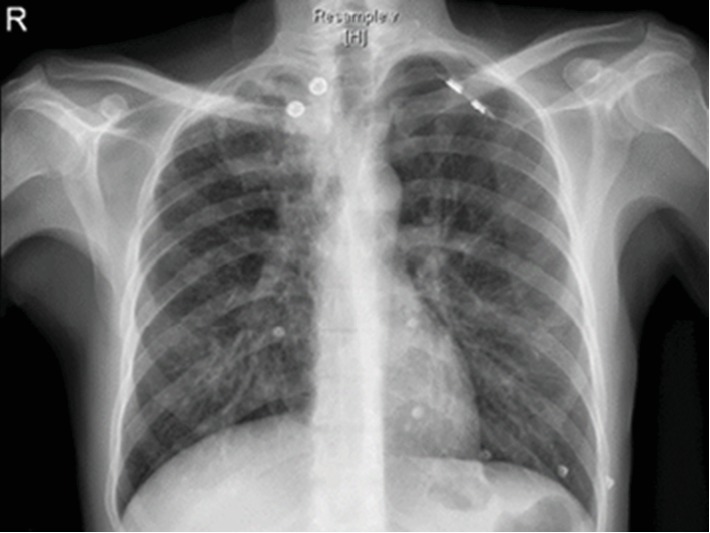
Chest radiograph, PA view showing cavitary lesion in the right upper lobe.
